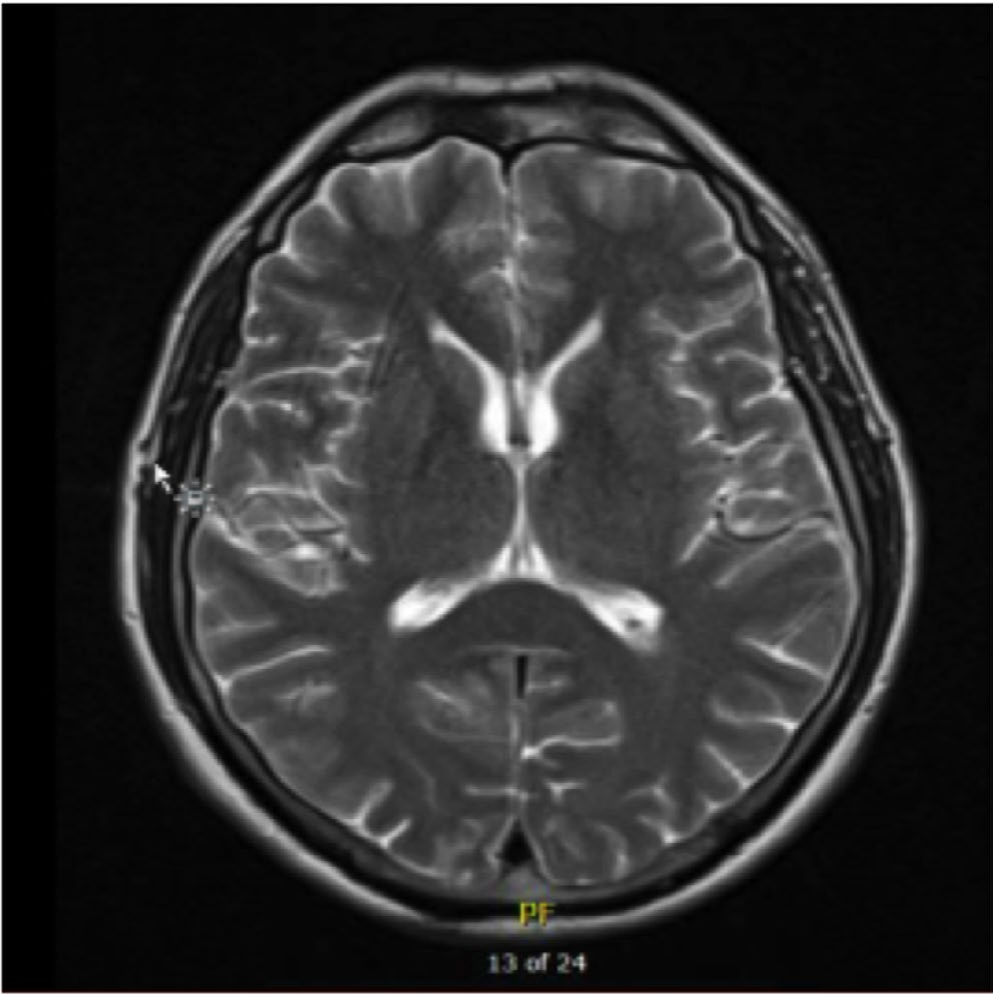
Transverse slice of initial MRI Head (T2 weighted) on first presentation. Normal scan
